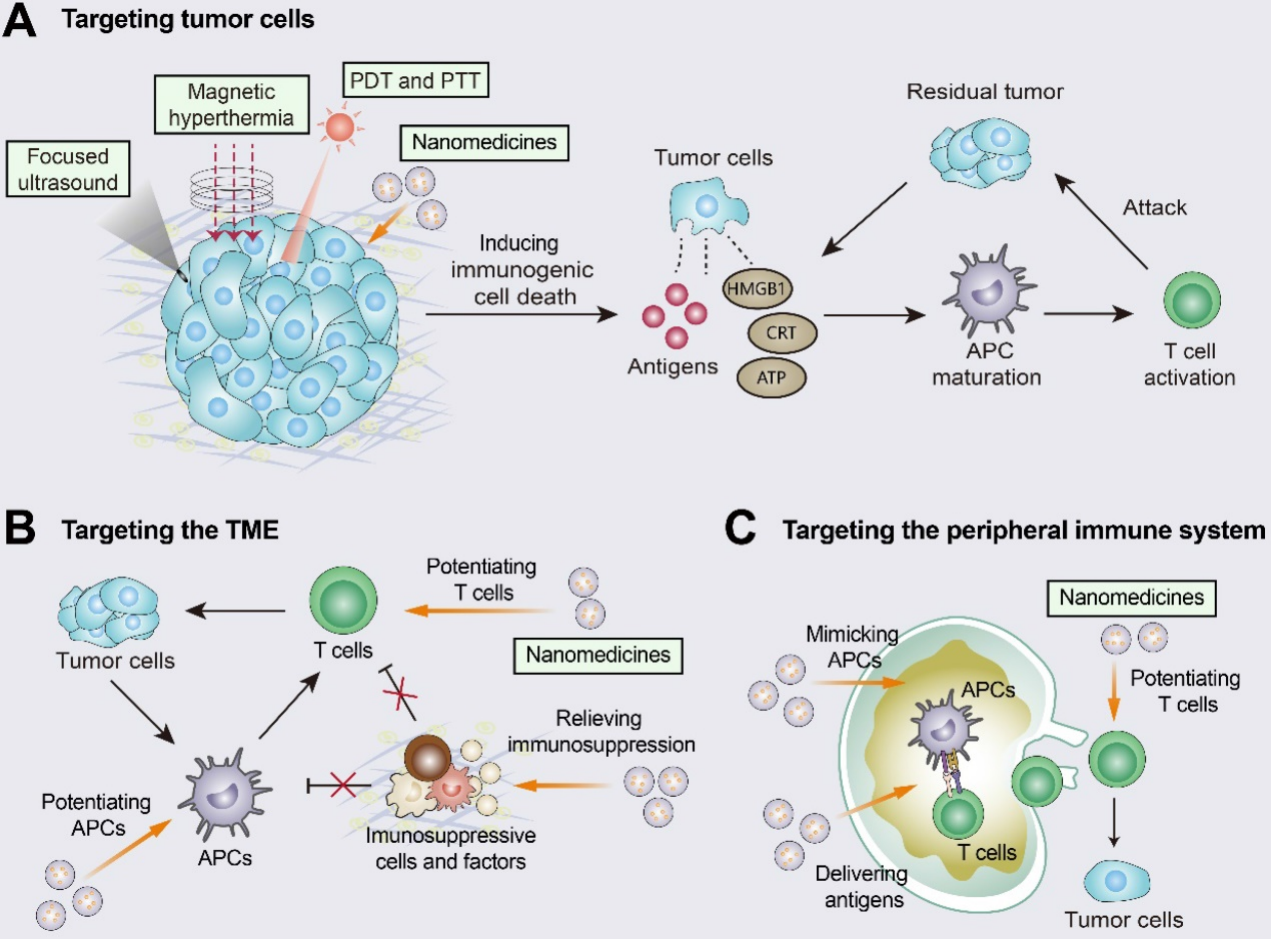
Improving T-cell infiltration with nanomedicines. Nanomedicines have three different targeting pathways: tumor cells, the TME, and the peripheral immune system. (A) Multiple approaches including photothermal therapy (PTT), photodynamic therapy (PDT), magnetic hyperthermia (MH), and high-intensity focused ultrasound (HIFU) can induce ICD by promoting the release of tumor antigens and damage-associated molecular patterns (DAMPs). The released DAMPs act as adjuvants to enhance the immunogenicity of the tumor and, together with the released tumor antigens, promote dendritic cell (DC) activation and T-cell priming. (B) When targeting the TME, nanomedicines inhibit immunosuppressive cells and immunosuppressive molecules (e.g., TGFβ) and enhance the activity of T cells. (C) When the peripheral immune system is targeted, nanomedicines are engineered to augment tumor antigen presentation and T-cell priming in lymph nodes. Adapted with permission from 150, copyright 2019 American Chemical Society.
